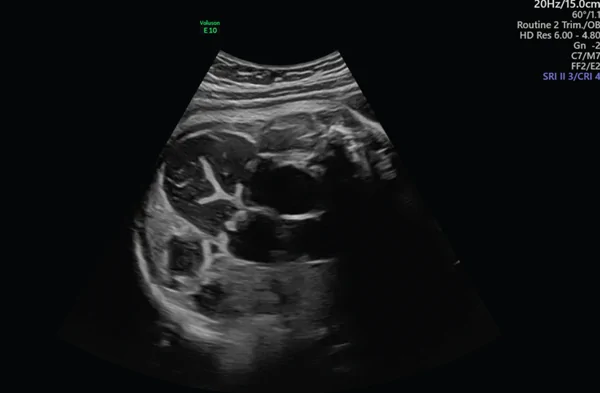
Case 2, dilated bowel loops at 36 weeks of gestation in patient with a MYO5B mutation (ultrasound image).
